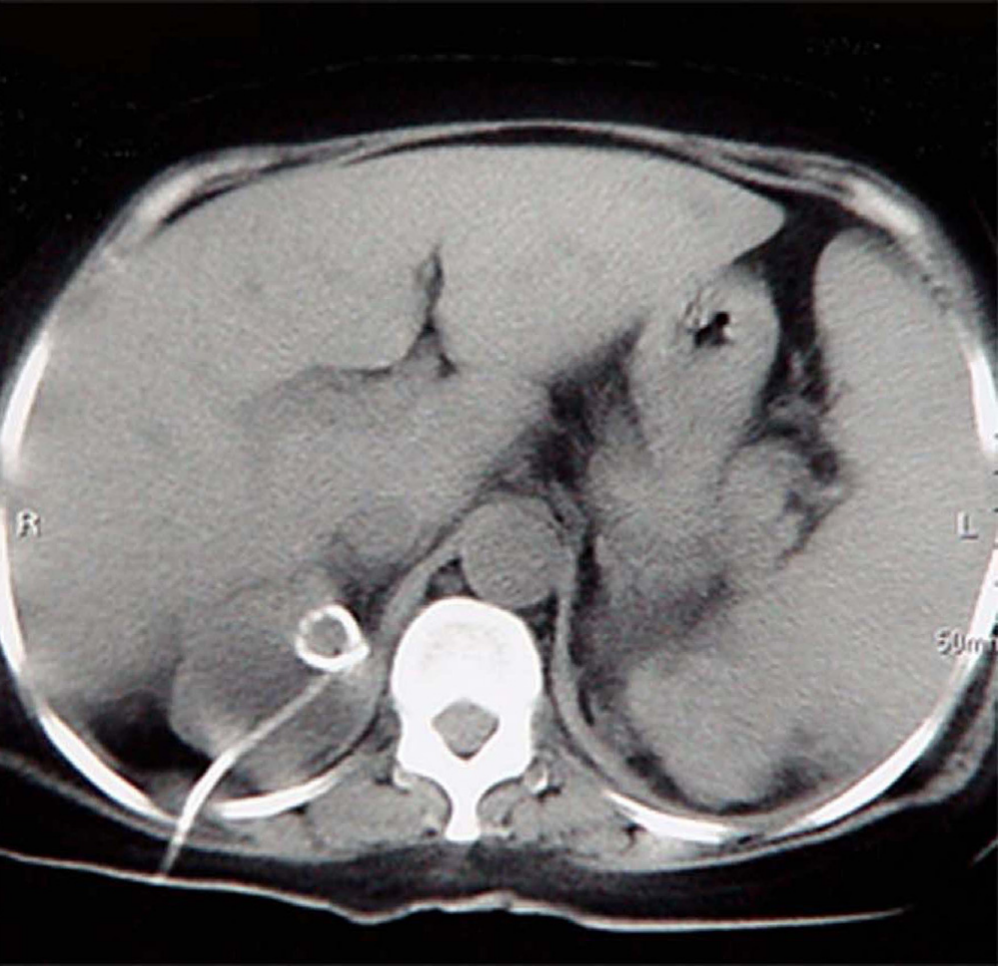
Axial CT scan of both kidneys without contrast demonstrates the nephrostomy catheter which was in the right kidney for further irrigation and drainage of the remaining pyocysts.
